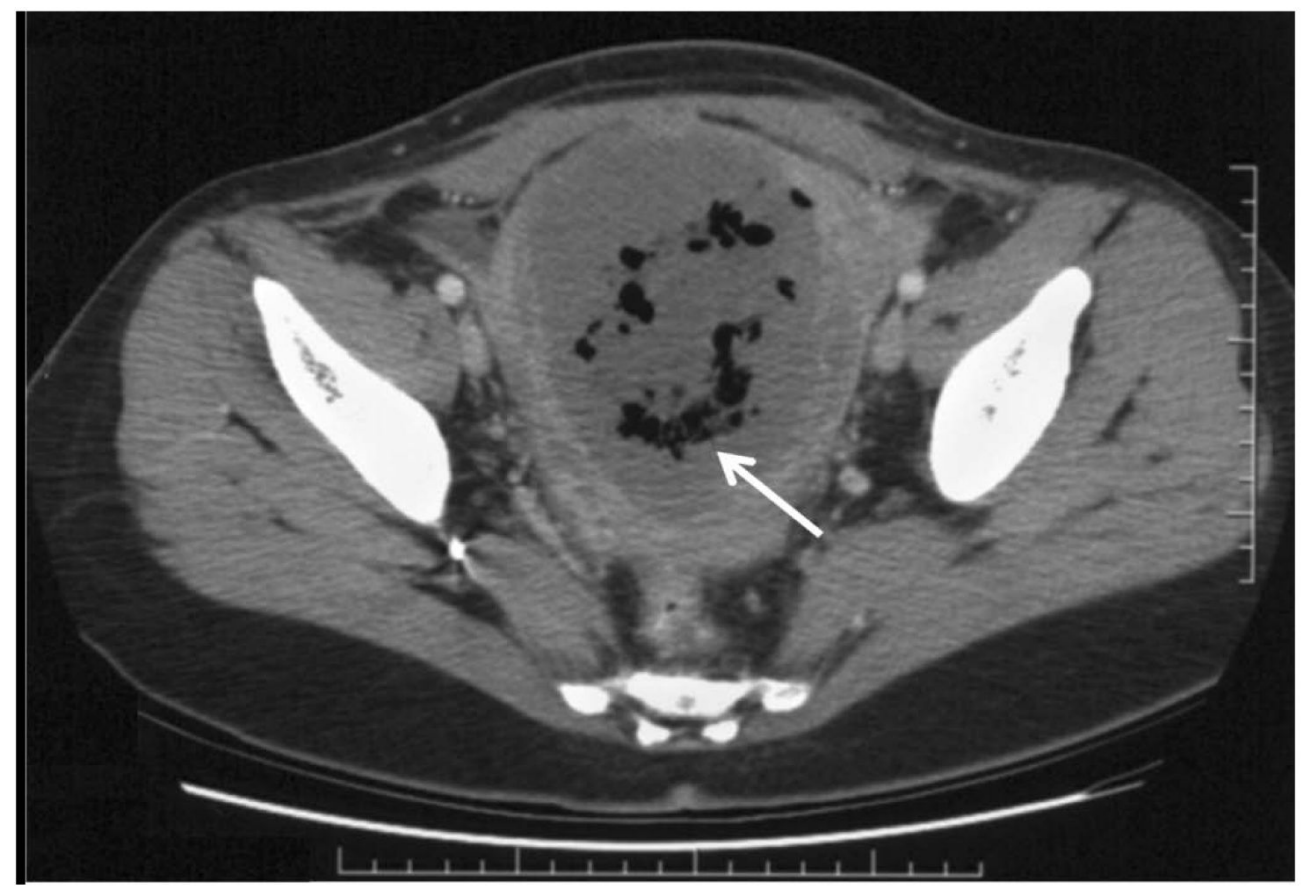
Computed tomography scan showing an enlarged uterus containing low attenuation tissue with significant amount of gas, consistent with uterine necrosis (arrow).
